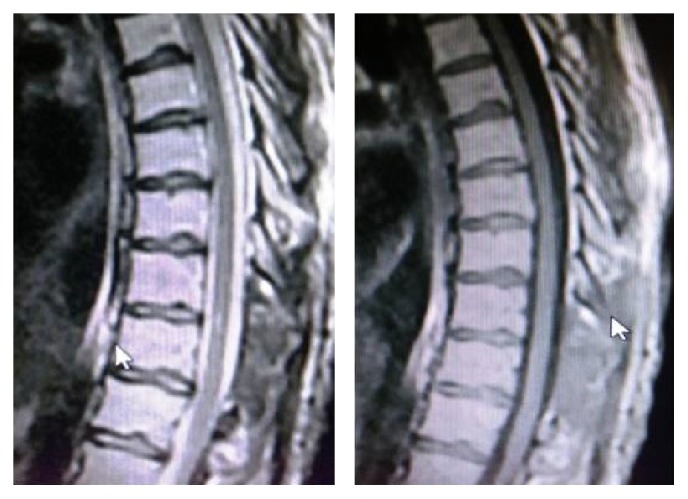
Postoperative thoracic sagittal T1-T2 MRI.
